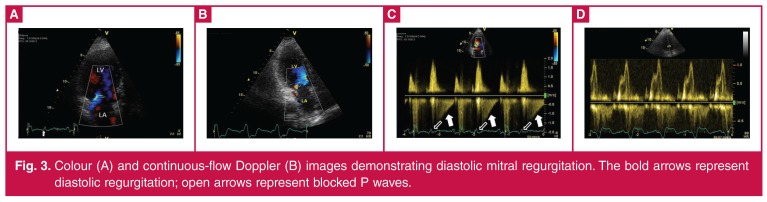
Colour (A) and continuous-flow Doppler (B) images demonstrating diastolic mitral regurgitation. The bold arrows represent diastolic regurgitation; open arrows represent blocked P waves.
